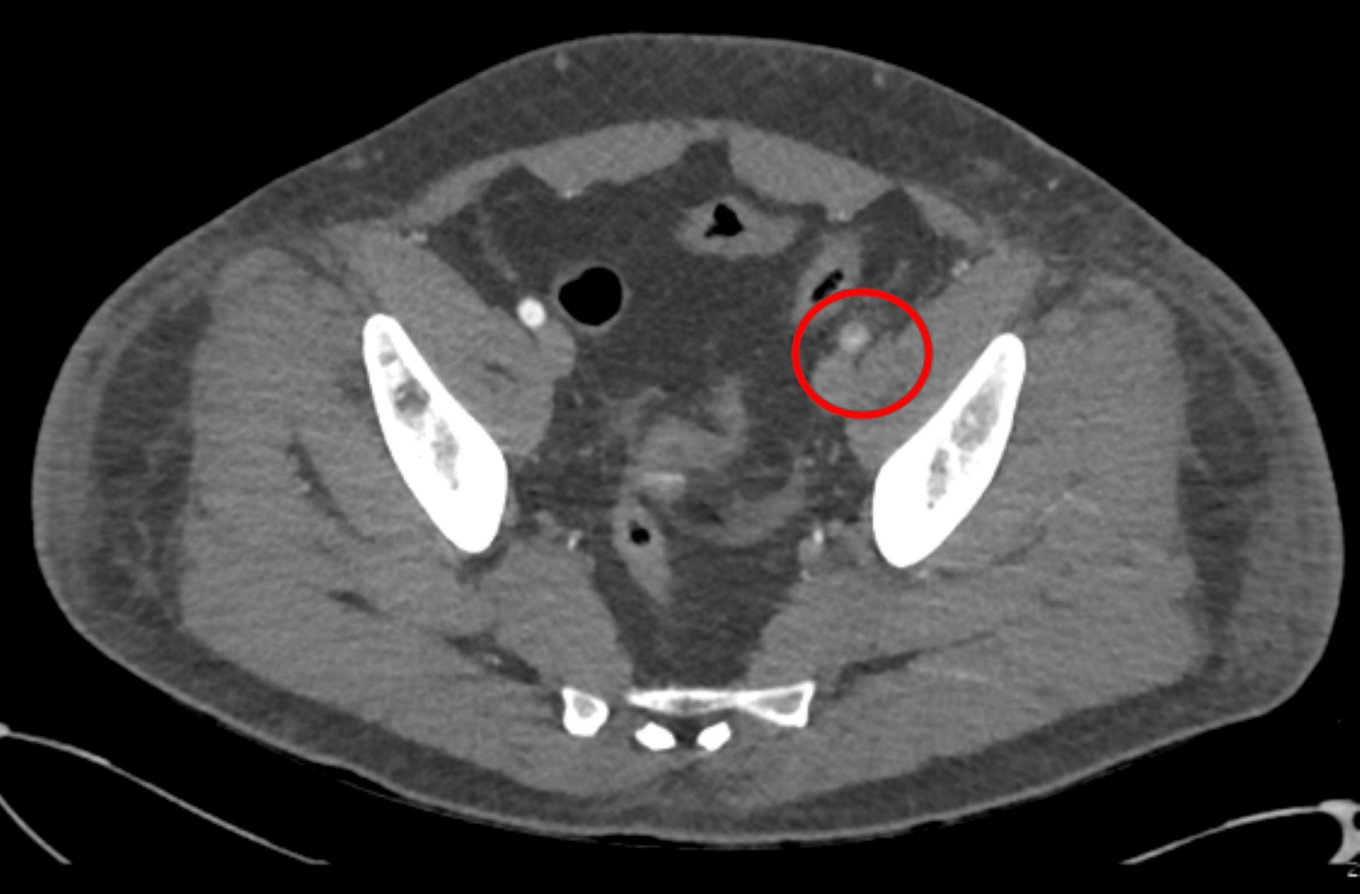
Repeat CT angiogram showing re-occlusion throughout the left limb CT: computed tomography
